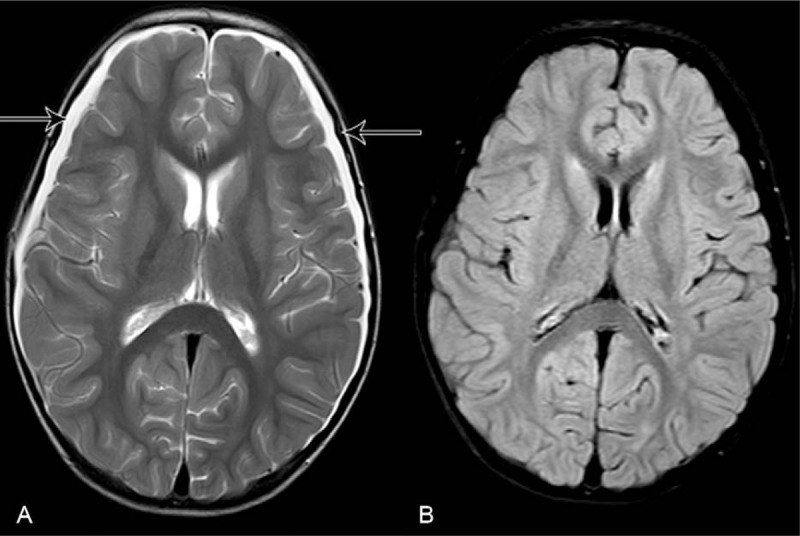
Cranial MRI showing bilateral subdural effusion on T2 (A) and the splenial lesion had completely disappeared on T2 (A) and FLAIR image (B). Hollow arrow showed bilateral subdural effusion in (A).
